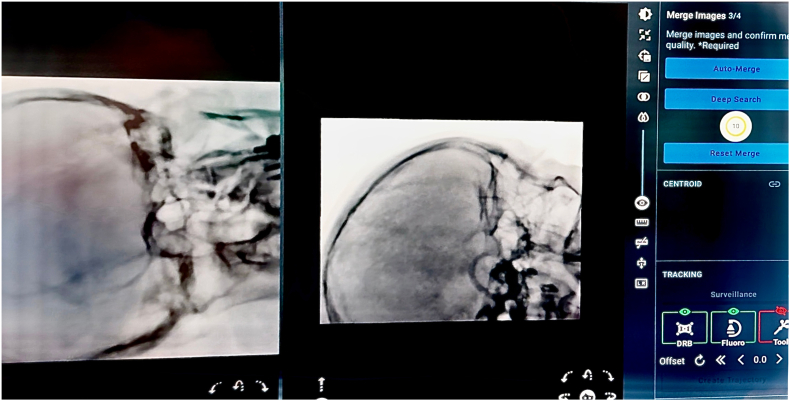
“Merge Images” fusion verification (ExcelsiusGPS). CT-to-fluoroscopy “Merge Images” verification screen on the ExcelsiusGPS platform, showing alignment of skull base bony landmarks and a merge quality score of 10/10, confirming accurate image fusion.
